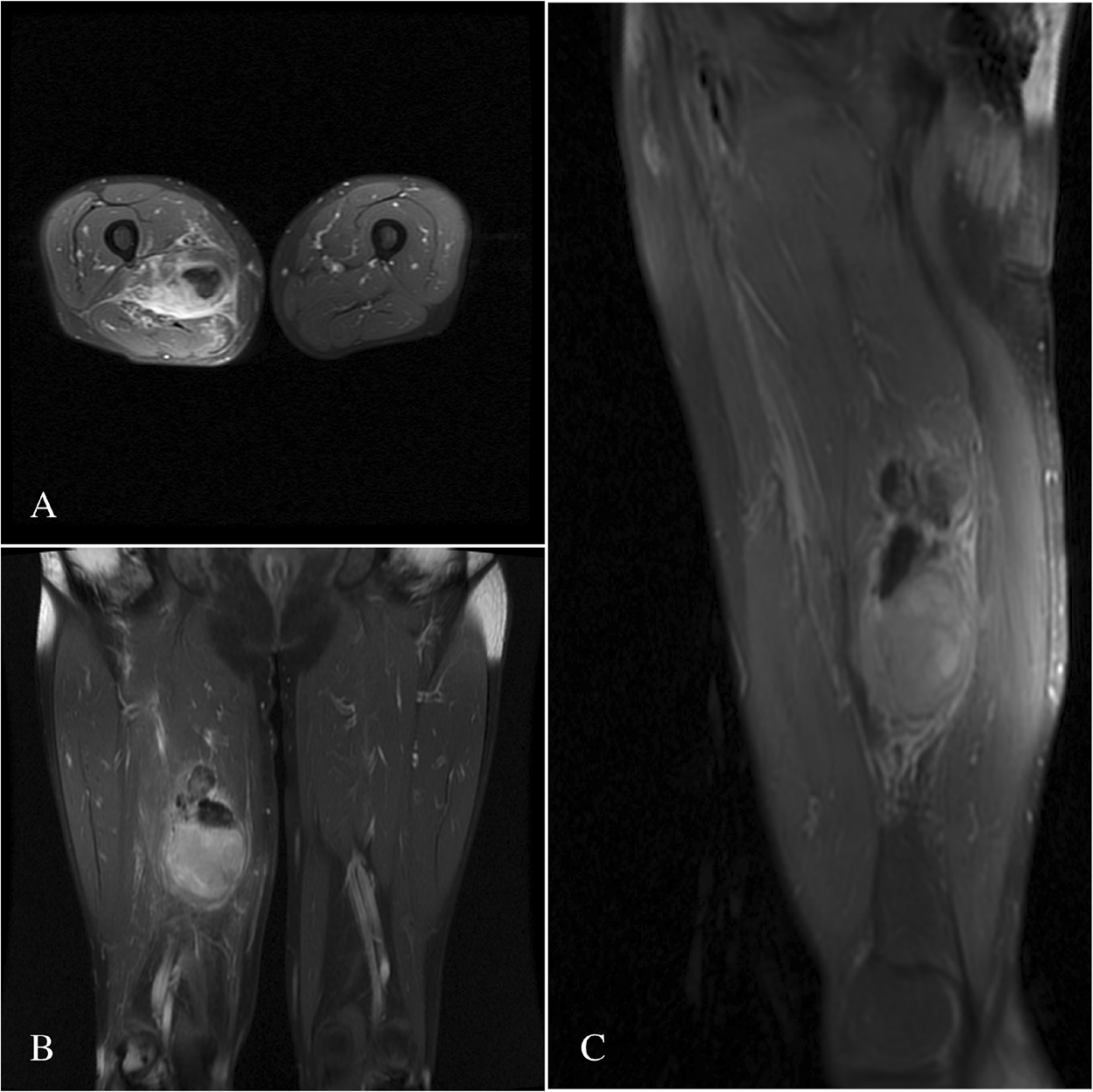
Enhanced T1-weighted and T2-weighted images in MRI for the lesion. Axial and coronal enhanced T1-weighted images show moderate enhancement (A, B). Sagittal T2-weighted image (C) shows an inhomogeneously isointense mass in the posterior thigh.
